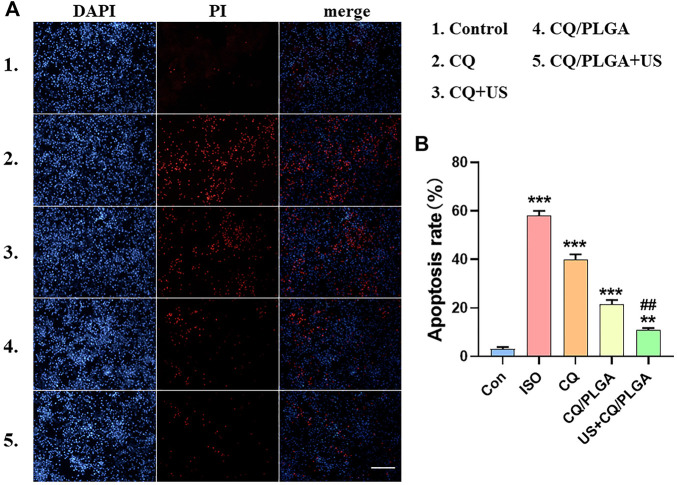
Evaluation of the inhibitory effect of ultrasound combined with CQ/PLGA on ferroptosis in HL-1 using fluorescent apoptosis detection. (A,B) When the fusion degree of HL-1 cells reached 80%, HL-1 cells were treated with different treatments (the control group did not do any treatment). After incubation for 24 h, the nuclei were counterstained with PI. The results of ImageJ analysis showed that ultrasound combined with CQ/PLGA could inhibit the apoptosis of HL-1 induced by ISO. The apoptosis rate of the ISO group was 58.08% ± 1.58%, and the apoptosis rate of the US + CQ/PLGA group was 10.90% ± 0.68% (vs. control group, *p* < 0.01, p* < 0.001, vs. other groups, ##*p* < 0.01, *n* = 5, Bar = 50 µm).
